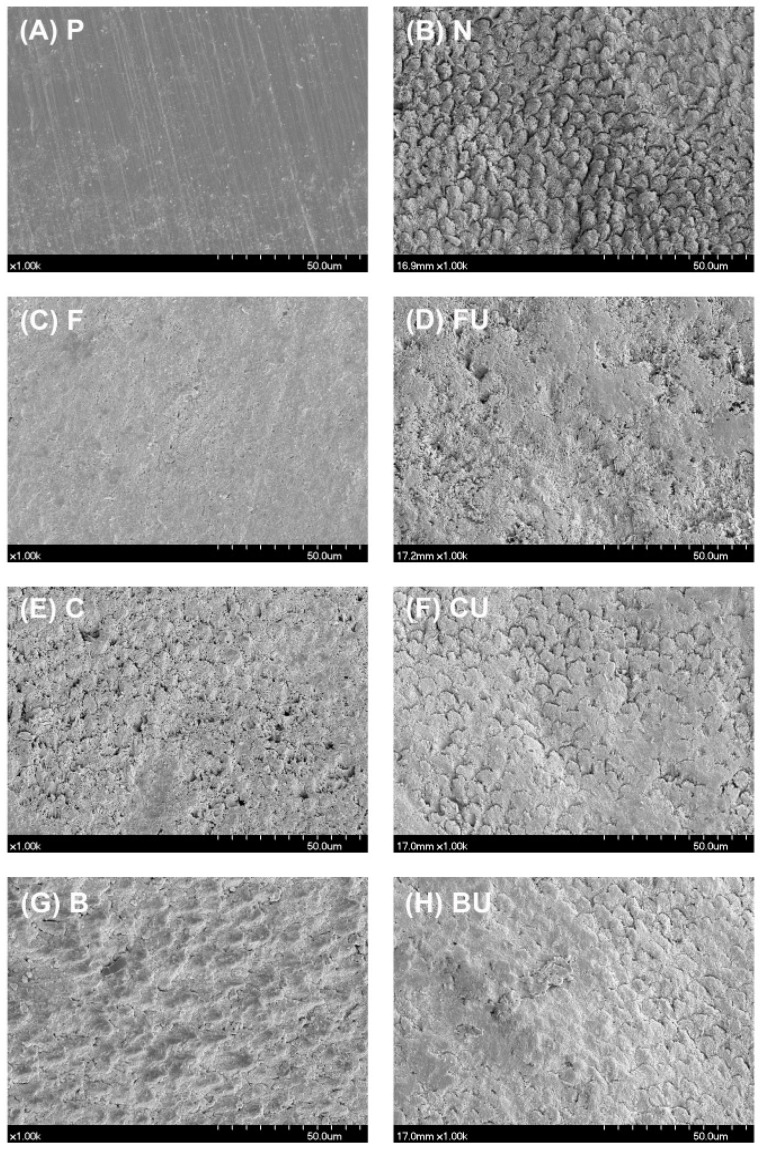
Representative FE-SEM images of all the experimental groups. (A) Group P, (B) Group N, (C) Group F, (D) Group FU, (E) Group C, (F) Group CU, (G) Group B, and (H) Group BU. Exposed enamel rods in Group N were covered with precipitates after the application of three varnishes with or without ultrasonication.
